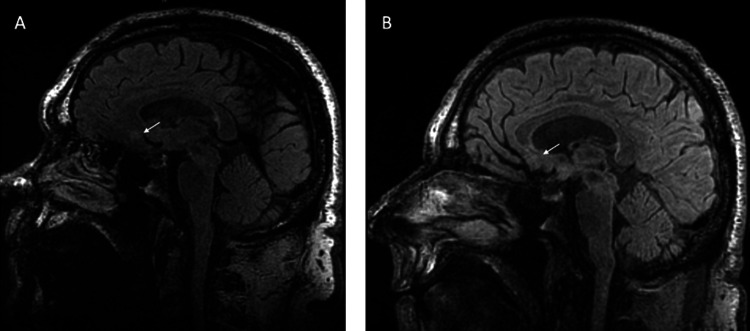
Subject 3 MRI brain at (A) six and (B) 21 months post-SRS The white arrows show the area of lesioning in the subgenual cingulate. SRS, stereotactic radiosurgery
